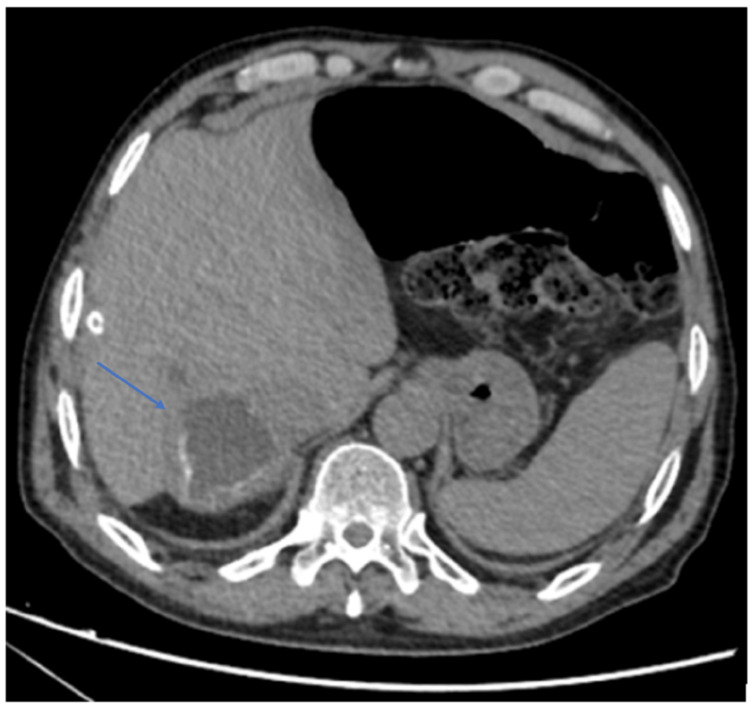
One-year follow-up abdominal CT scan CT: computed tomography
